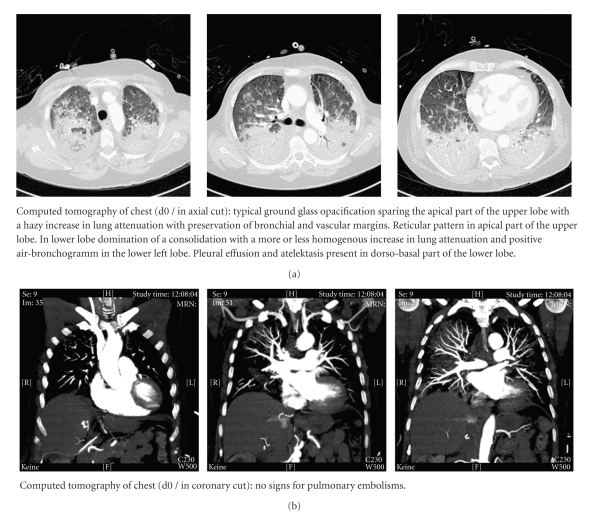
Coronary and axial cuts of the computed tomography of the chest immediately after the end of surgery before patient's admission to the intensive care unit. d: day.
